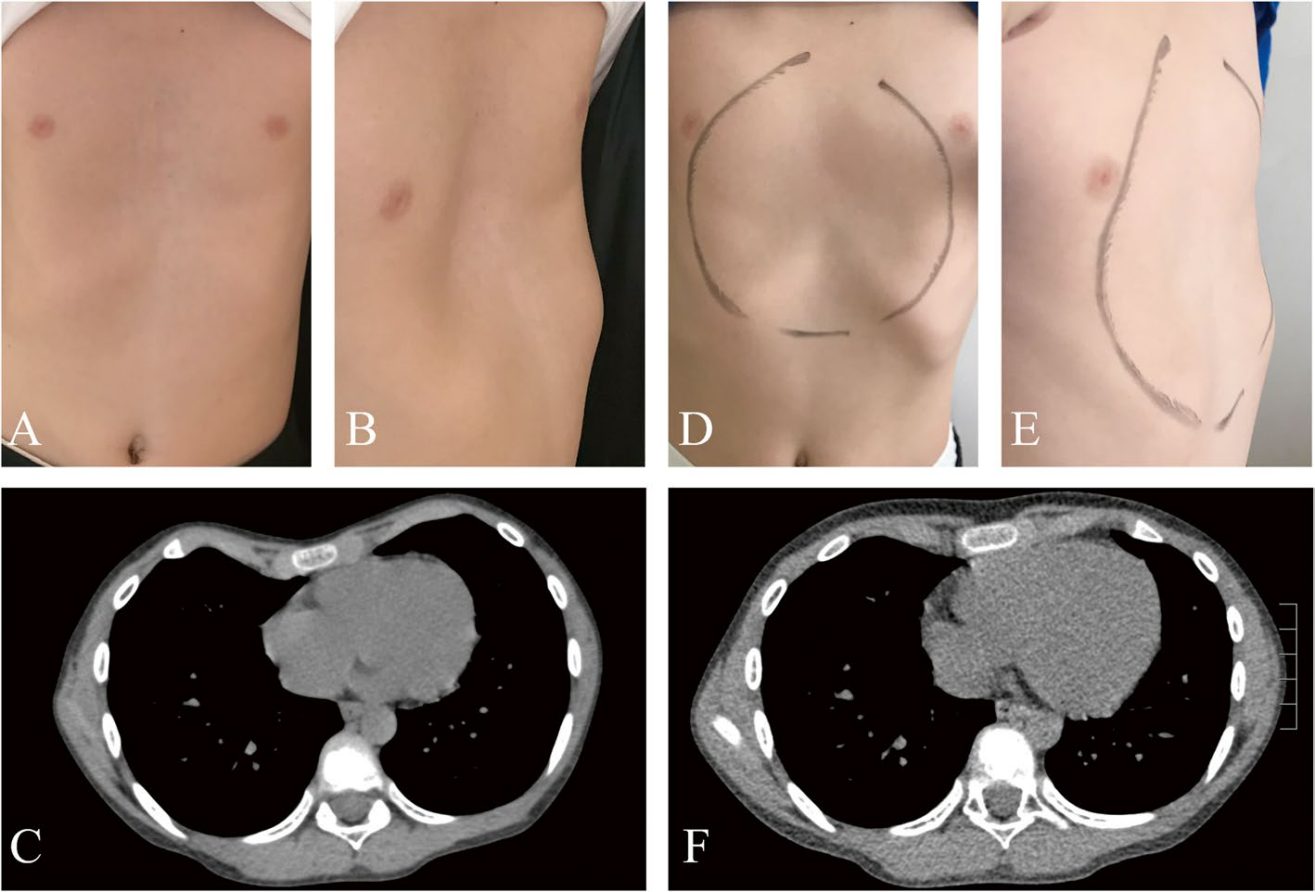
Evaluation of treatment outcomes after vacuum bell application. Pictures and chest computed tomography (CT) were taken from a 9-year-old male patient just before starting treatment (A, B, C) and after vacuum bell therapy (D, E, F). A Anterior view before vacuum bell application; B Lateral view before vacuum bell application; C Chest computed tomography taken before starting therapy; D Anterior view after treatment; E Lateral view after treatment; F Chest computed tomography taken after treatment
